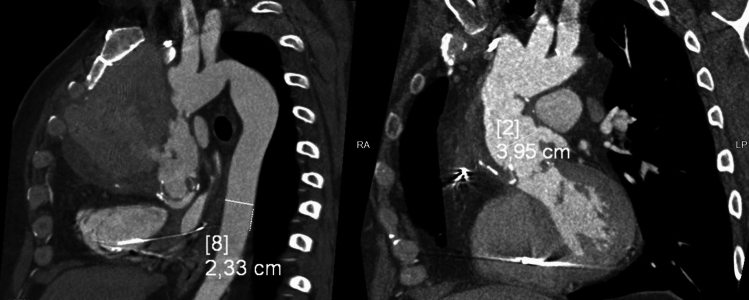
CT scan before and after reoperation
